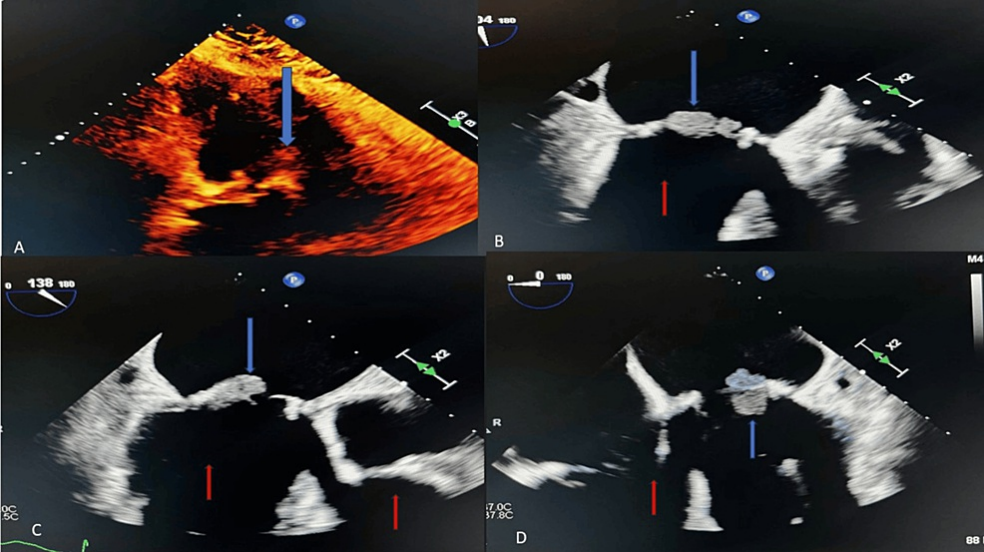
Echocardiographic image showing mobile vegetation (blue arrows) on the anterior mitral leaflet and moderately dilated right and left atriums (red arrows).
